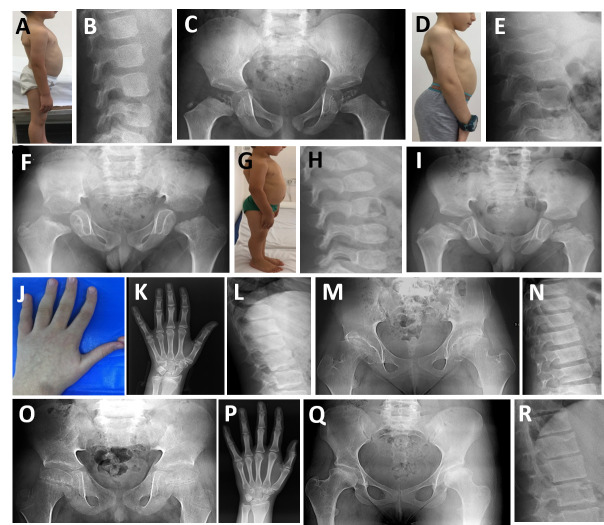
Patients with monoallelic disease-causing variants in *COL2A1*. P1 at age 4.5 (A–C), P2 at 7 (D–F), and P3 at 3.5 years of age (G–I). Note marked lumbar lordosis (A,D,G), mildly ovoid vertebrae (B,H), irregular vertebral endplates (E), and small, fragmented, irregular, or destroyed femoral heads with slightly broad and irregular proximal femoral metaphyses (C,F,I). P4, with SED and metatarsal shortening, at age 16: ulnar deviation of the second finger (J), normal metacarpal and phalangeal bones (K), slightly elongated vertebrae with irregular endplates (L), and irregularities of the femoral head and acetabulum with narrowing of the joint space and coxa valga (M). P5, with SED Stanescu type, at age 7, had mild platyspondyly (N), an irregular acetabulum, a slightly flattened femoral head, an irregular proximal femoral metaphysis, a broad femoral neck, and coxa valga (O) on radiographs. P6, with Stickler syndrome type I, at age 17, had long metacarpal bones (P), flat and irregular epiphyses of the femoral head (Q), and rectangular vertebral bodies (R).
